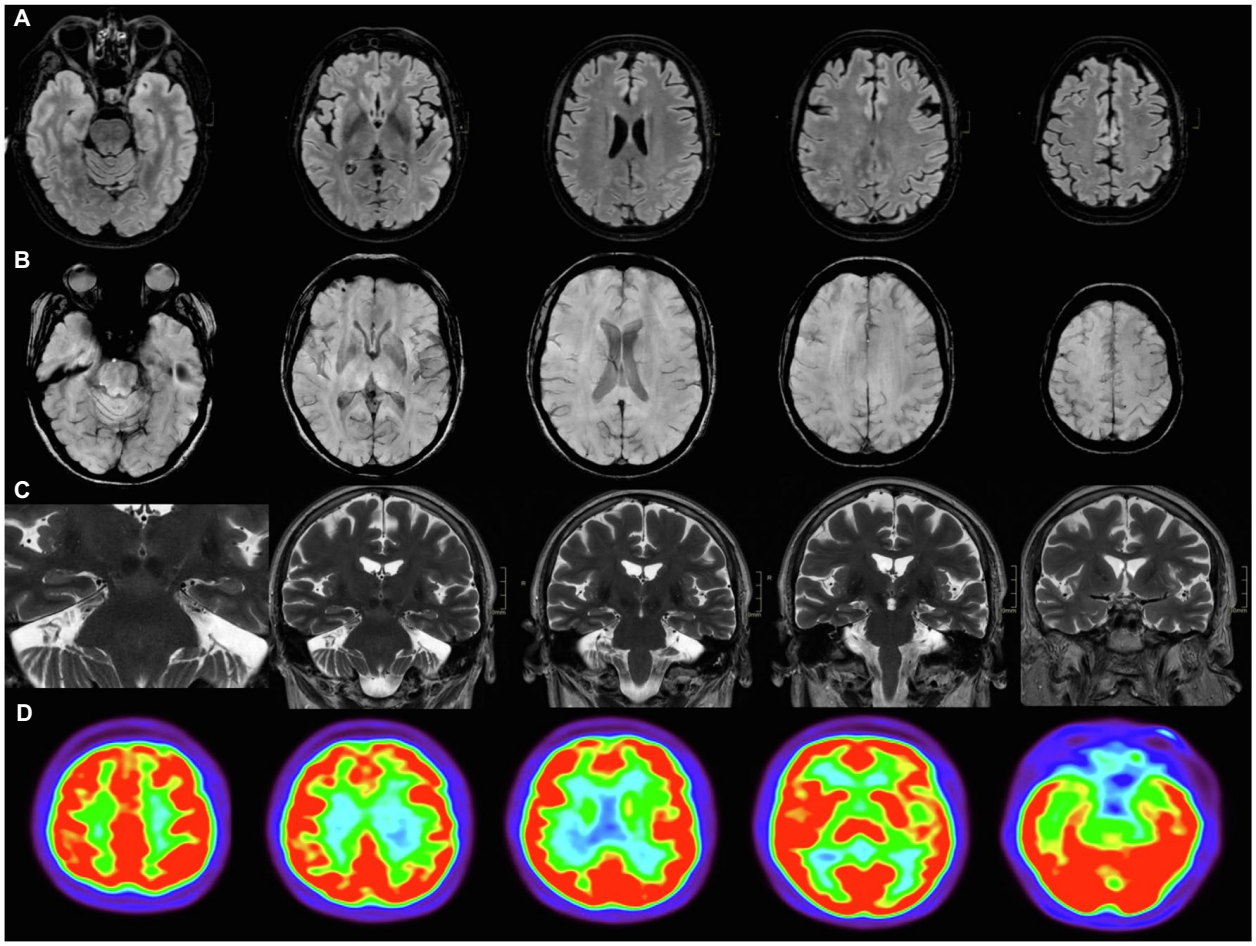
Neuroimaging. Brain MRI (A–C) and FDG-PET (D). (A) Axial FLAIR, with no white matter hyperintensities; (B) axial SWAN, showing no microbleeds; (C) coronal FSE T2 PROPELLER, with no hippocampus atrophy or ischemic lesions; and (D) FDG-PET showed no regions of hypometabolism visually.
